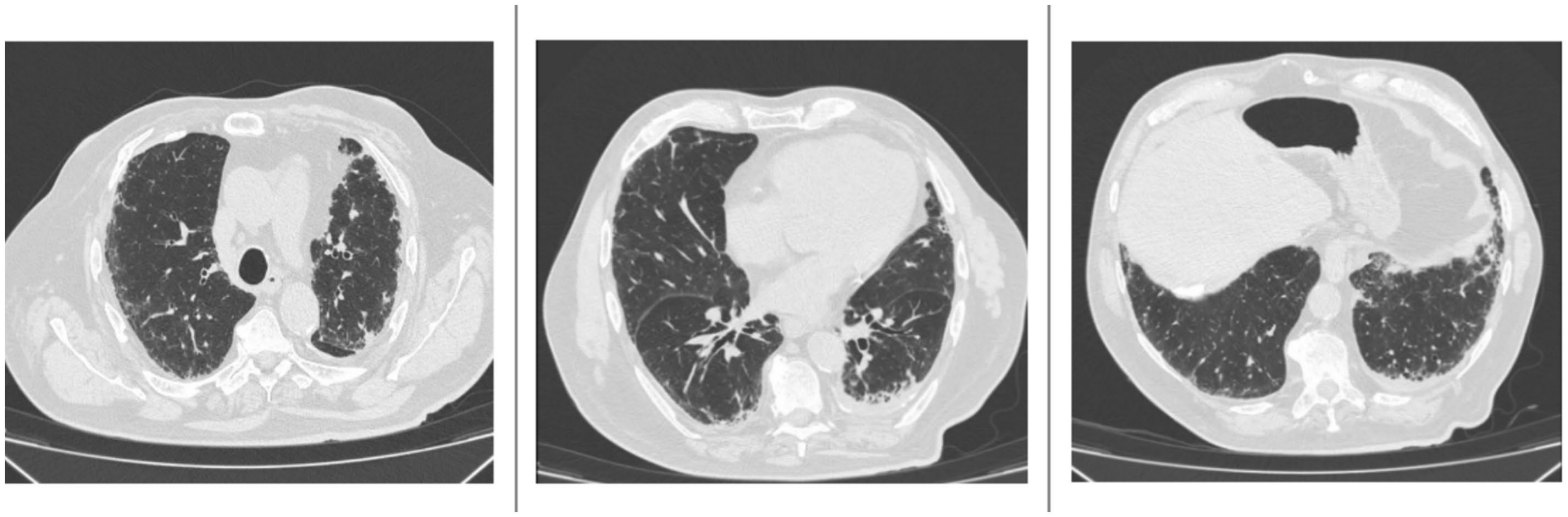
High-resolution computed tomography (HRCT) scans performed in June 2024 show multiple millimetric subpleural bronchiolectases affecting both lower lobes, the lingula, and the middle lobe. There is also a concomitant diffuse fine interstitial reticular thickening, which is also present in the upper lobes.
